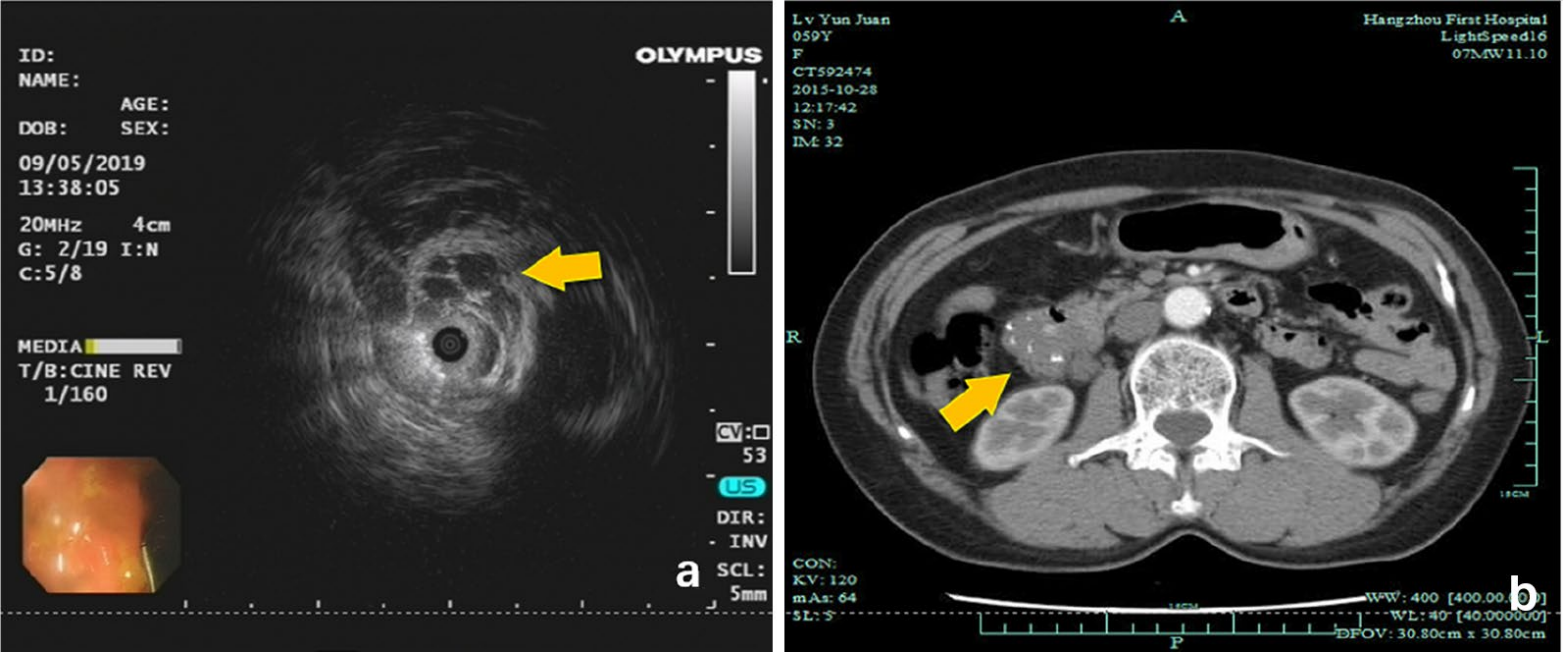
Supplement endoscopic pictures, in order to show imaging. a: HL of the ileocecal vasculature, Endoscopic ultrasound Endoscopic Ultrasound (EUS) showed that the hypoechoic shadows originated from the mucosal muscle layer and the submucosal layer, revealing an oval shape and internally separated (Fig. 2a, EUS); (b) Duodenum HL, Computed tomography (CT) of an angioma showed a low-density mass in the lower part of the duodenum, with multiple speckle-like high-density shadows; the size of the lesion was approximately 4.4 × 2.7 cm. Contrast-enhanced CT showed continuous uneven enhancement.
